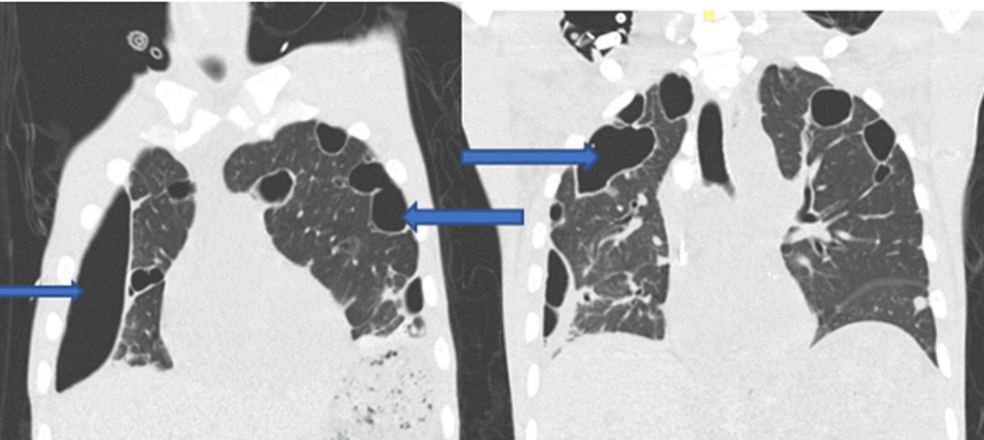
Computed tomography (CT) scan of the chest. The blue arrows identify the loculated right-sided pneumothorax and multiple pneumatoceles.
